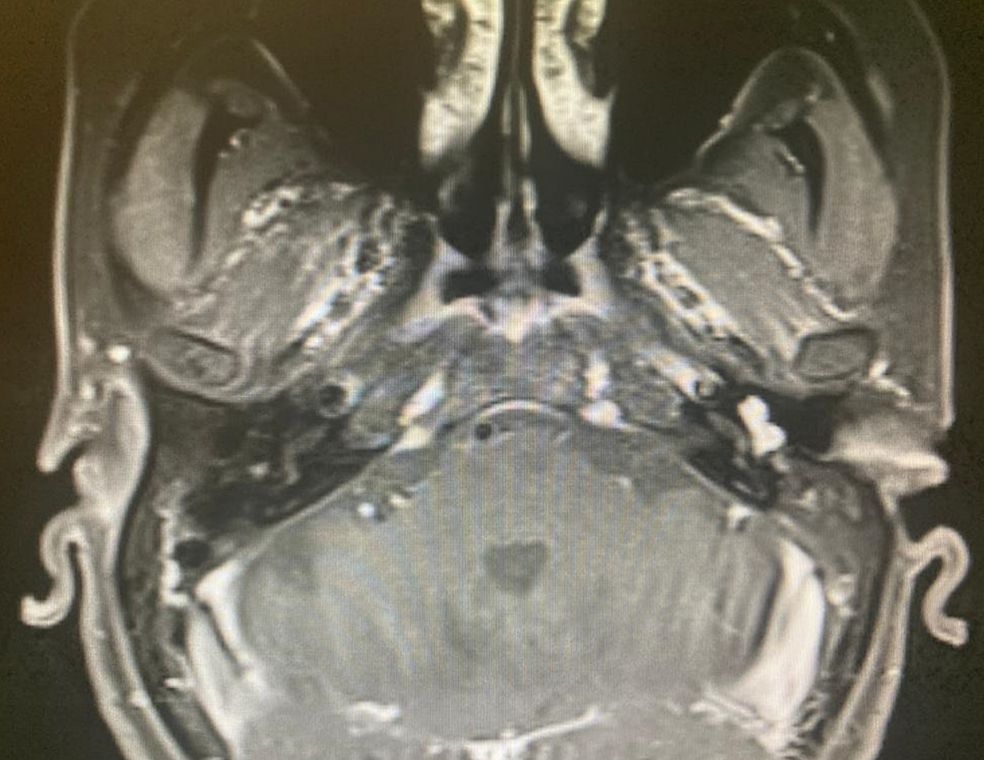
MRI of temporal bone showing the mass filling the mesotympanum
